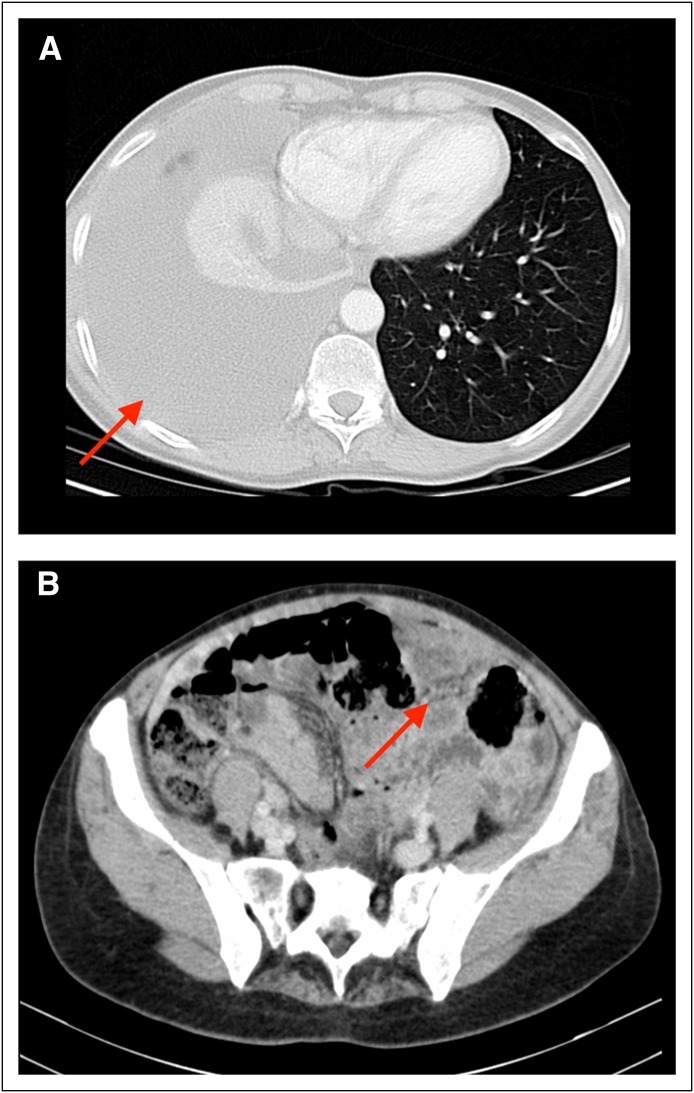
Computed tomography images of the chest and abdomen. (A) Right-sided pleural effusion with right lower lobe atelectasis (arrow). (B) Omental thickening in the left flank (arrow).
